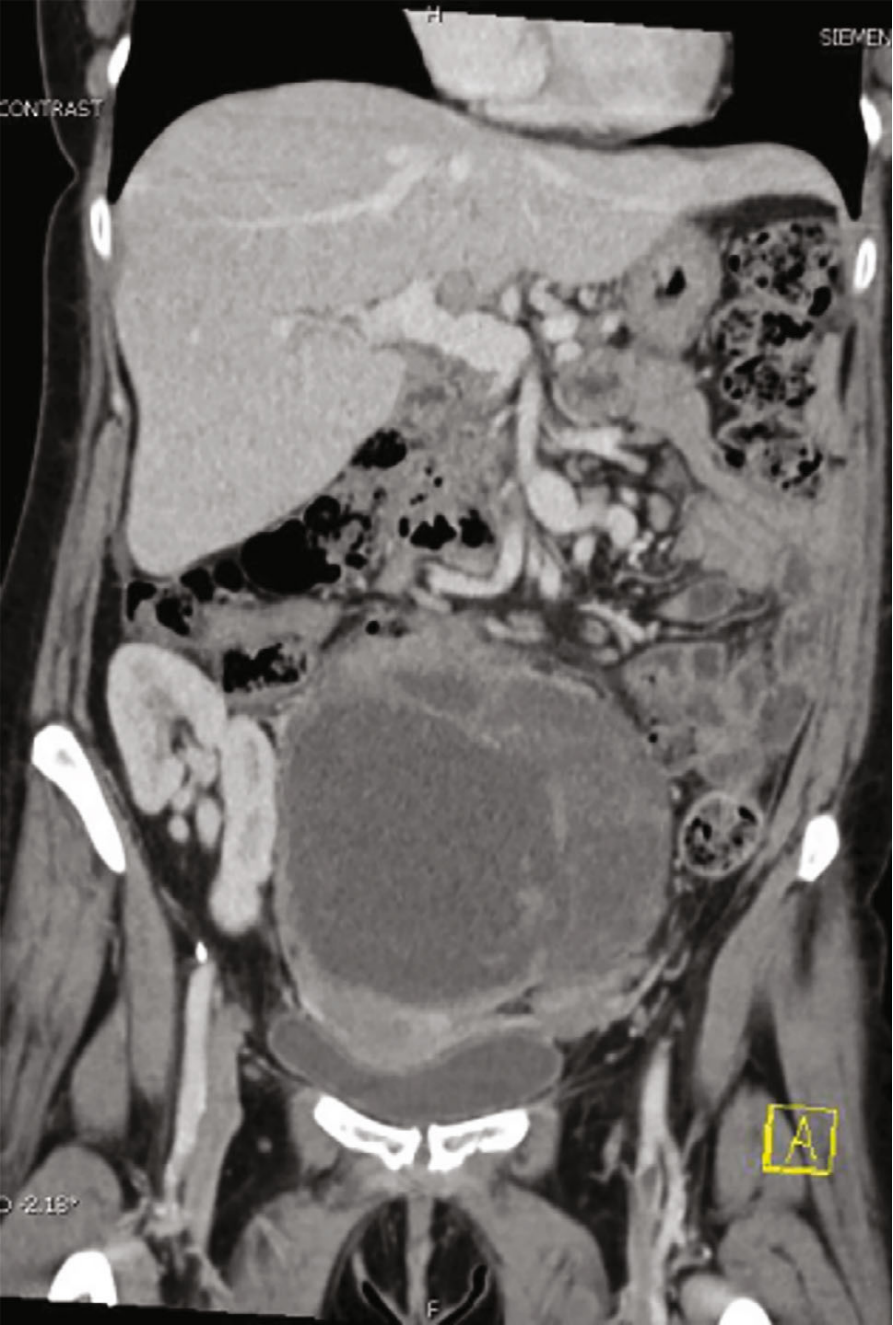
Coronal image of CT scan demonstrating large midline lower abdomen/pelvic mass with cystic areas measuring up to 19 × 12 × 13 cm, with mass effect on pelvic organs and bowel loops.
